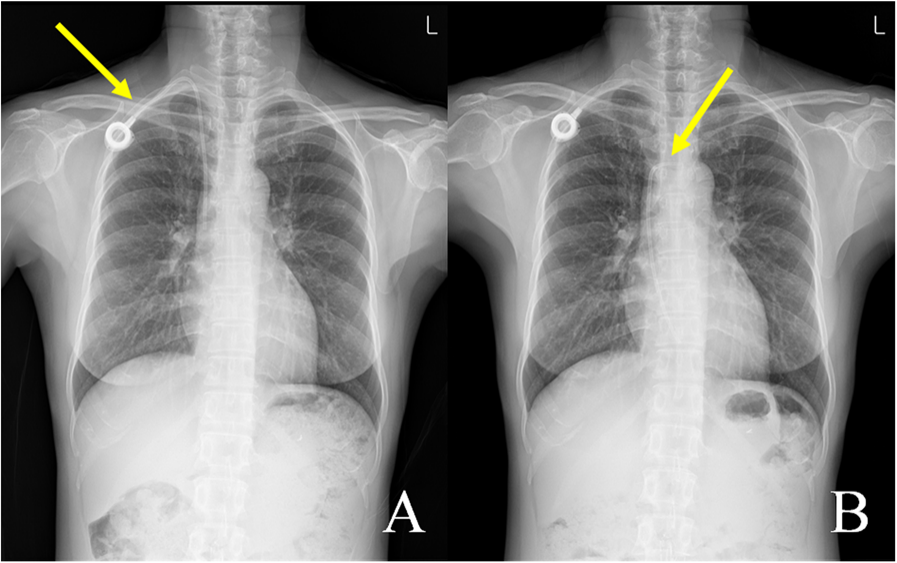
A totally implantable venous access port (TIVAP) on chest radiography. a Chest radiography shows a TIVAP locates at a appropriate position after TIVAP placement procedure. Arrow indicates a TIVAP. b Chest radiography shows fracture and migration of the catheter of a TIVAP. Arrow indicated the fractured catheter fragment moved into right atrium
